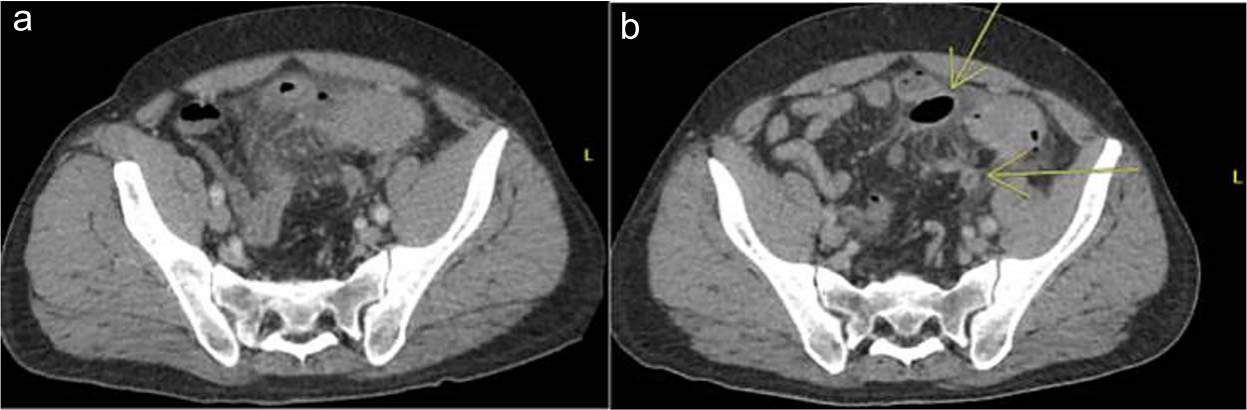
Patient B. a. Initial CT abdomen and pelvis showing acute diverticulitis with small adjacent abscess. b. Repeat CT abdomen and pelvis with worsening complicated diverticulitis.
